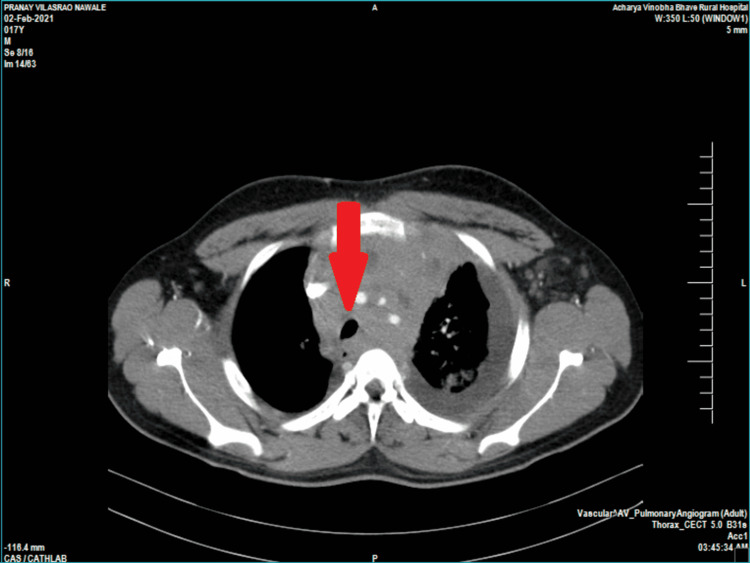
CT depicting isodense mass in the paratracheal region (arrow) CT: computed tomography
